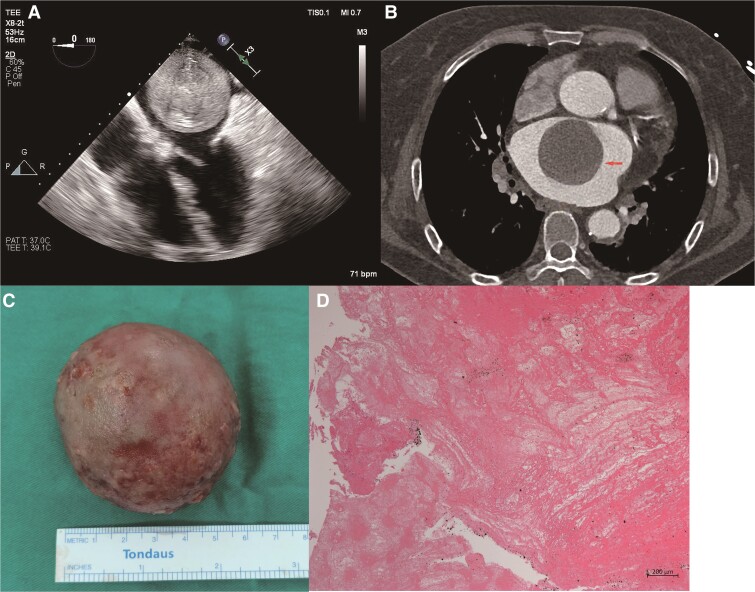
(*A*) Transoesophageal ultrasound detected a 58 × 55 mm moveable spherical mass in the left atrium. (*B*) Computed tomography showed a giant mass in the left atrium (the arrow shows the mass in the left atrium). (*C*) The giant left atrial mass is surgically removed. (*D*) Pathological examination confirmed the mass was a thrombus.
